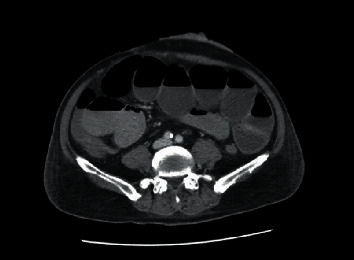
CT of the abdomen showing the small bowel obstruction with transition zone at the proximal ileum.
